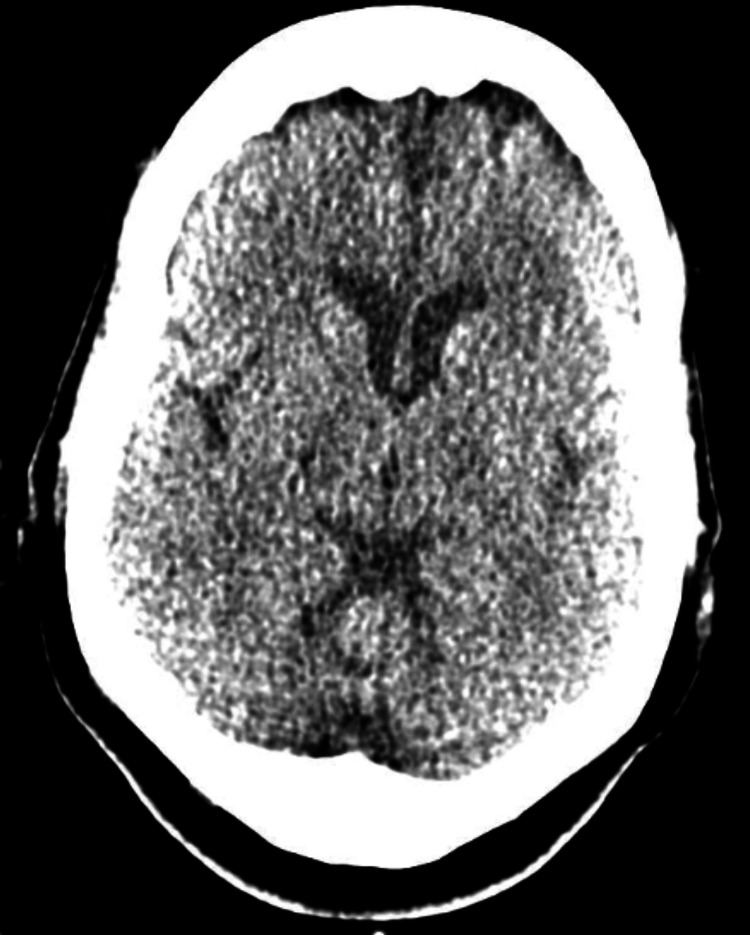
Non-contrast CT brain with no remarkable identified pathology.
